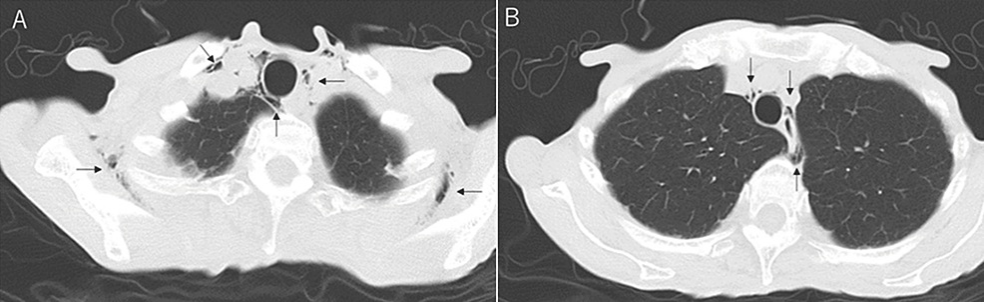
Chest computed tomography The image near the lung apex shows free air in the mediastinum and subcutaneous emphysema in the soft tissues of the back (A). Free air can be seen from the spine to the mediastinum surrounding the bronchi (B).
